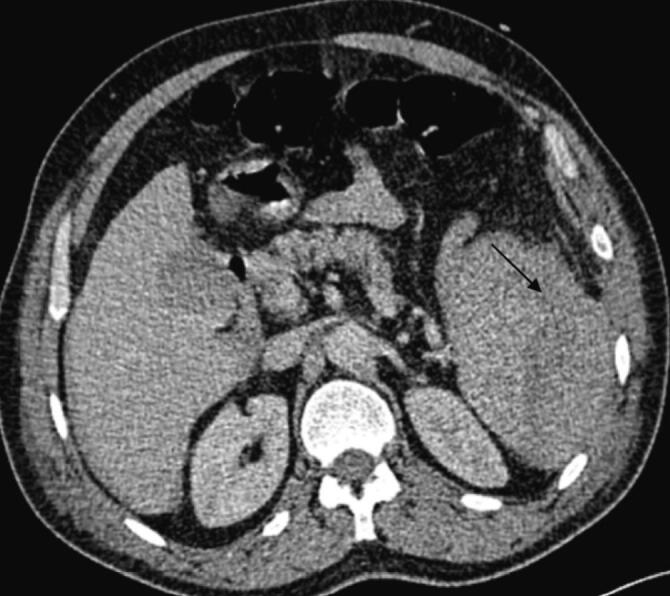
Axial section of the CT scan showing a subcapsular haematoma of the spleen.
